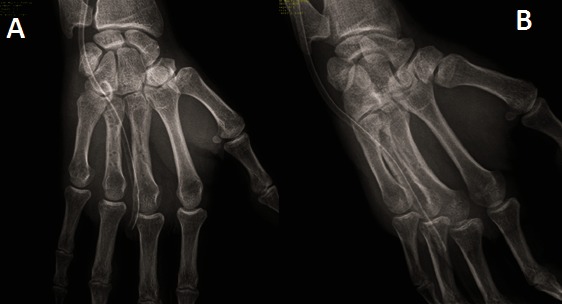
Postoperative plain (A) and oblique (B) radiographs
